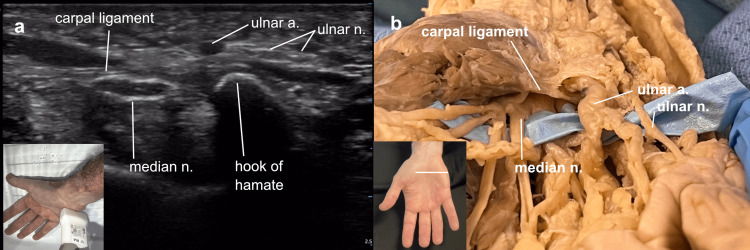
Ulnar (Guyon’s) canal Ulnar (Guyon’s) canal: (a) The ultrasound view of a right hand obtained in a water bath to avoid air artifact demonstrates the ulnar canal superficial to the transverse carpal ligament, (b) the cadaver view demonstrating the degree to which the ulnar canal overlies the carpal tunnel.
